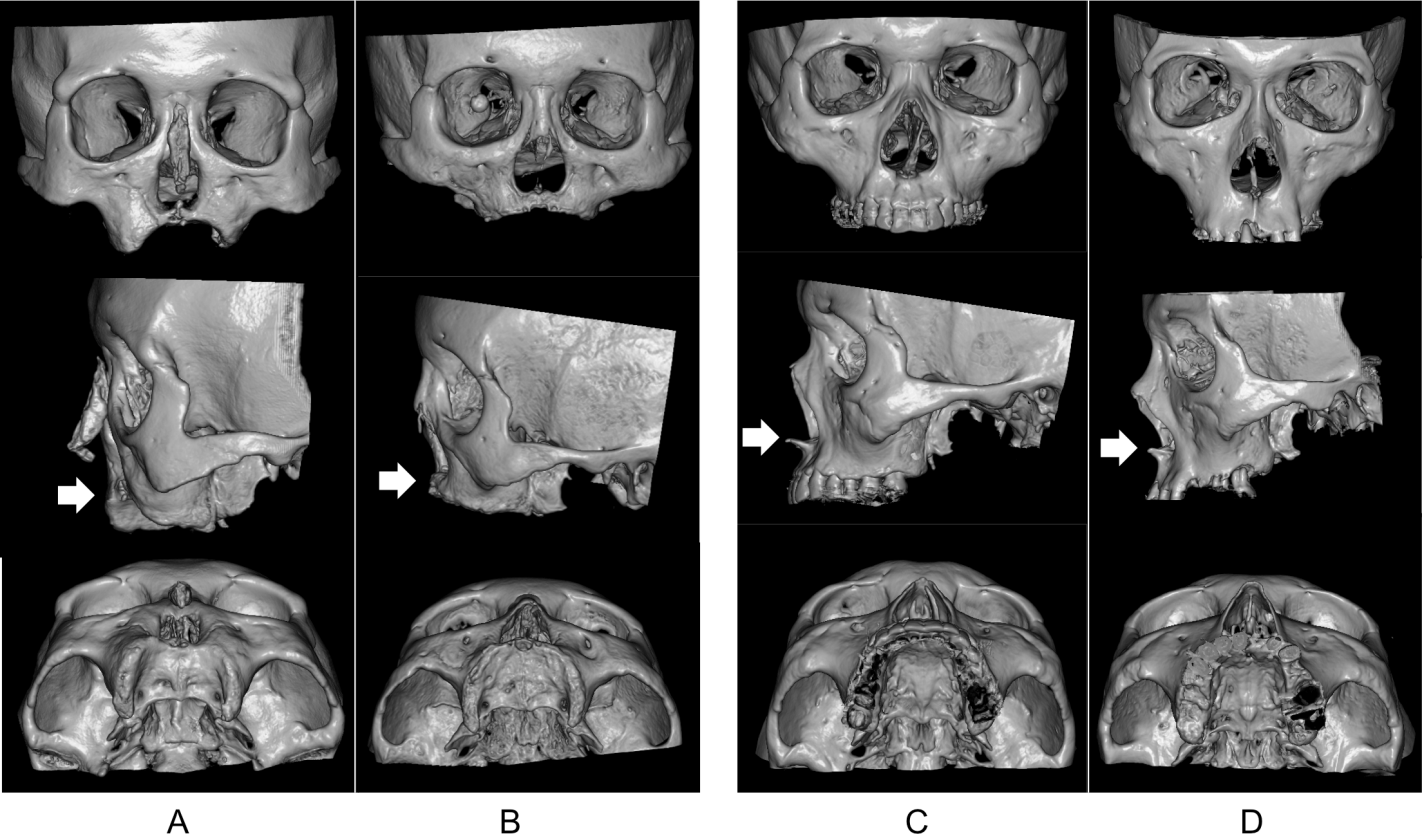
Reconstructed stereoscopic CT images of the maxilla. Two severely deformed cases, A (ID 9) and B (ID12), and two control cases, C (ID C1) and D (ID C3), are shown. The front, left side, and bottom view of the cranium are shown from top to bottom. The deformities of the anterior nasal spine and the alveolar process are easily observed in A and B. The result is that the retracted surface of the central maxilla and the regression of the alveolar process look nearly symmetric and crescentic. Arrows indicate the anterior nasal spine (ANS) or the corresponding point.
